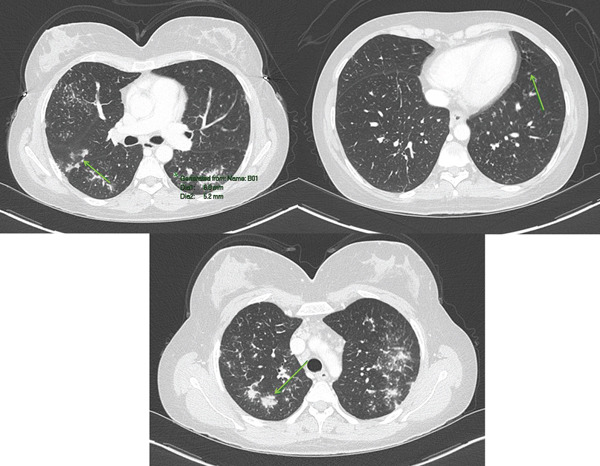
Axial lung‐window chest CT images, demonstrating multiple peribronchovascular and perilymphatic nodular opacities.
